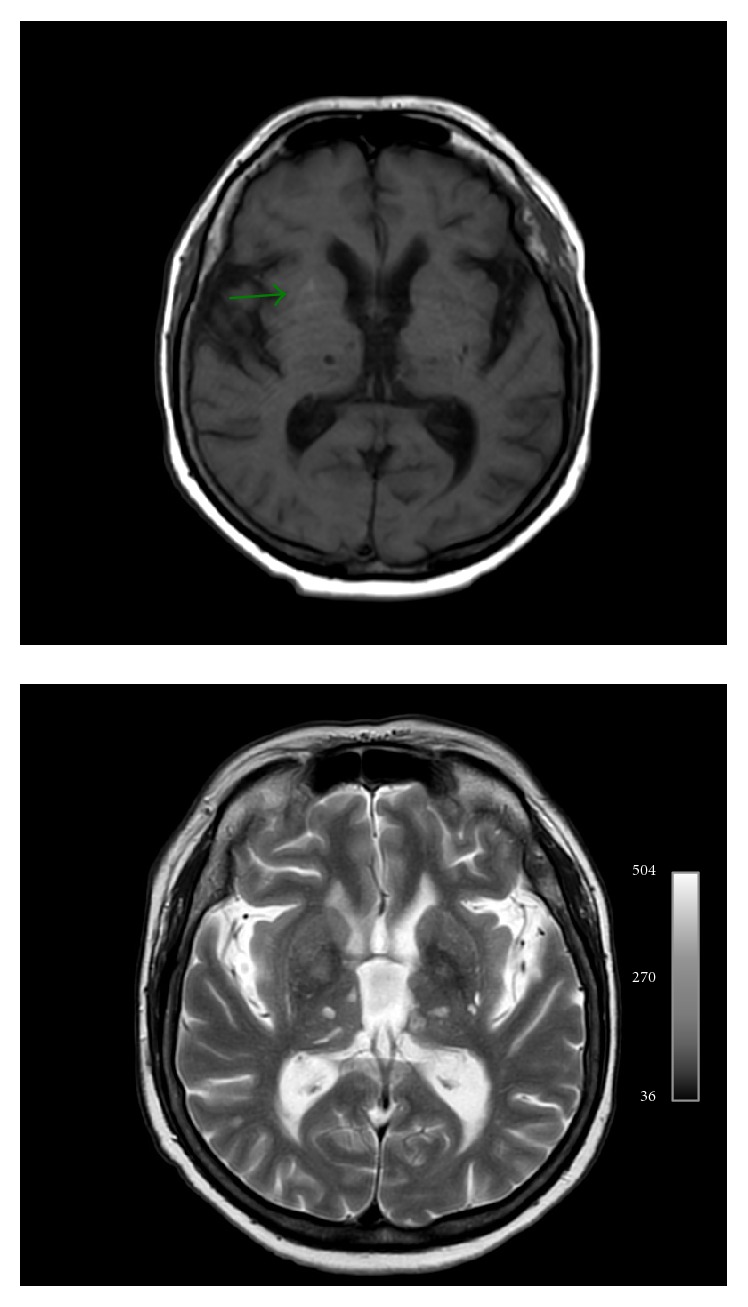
Axial T1-weighted sequence depicts right basal ganglia hyperintensity with unremarkable signal abnormality on axial T2-weighted.
